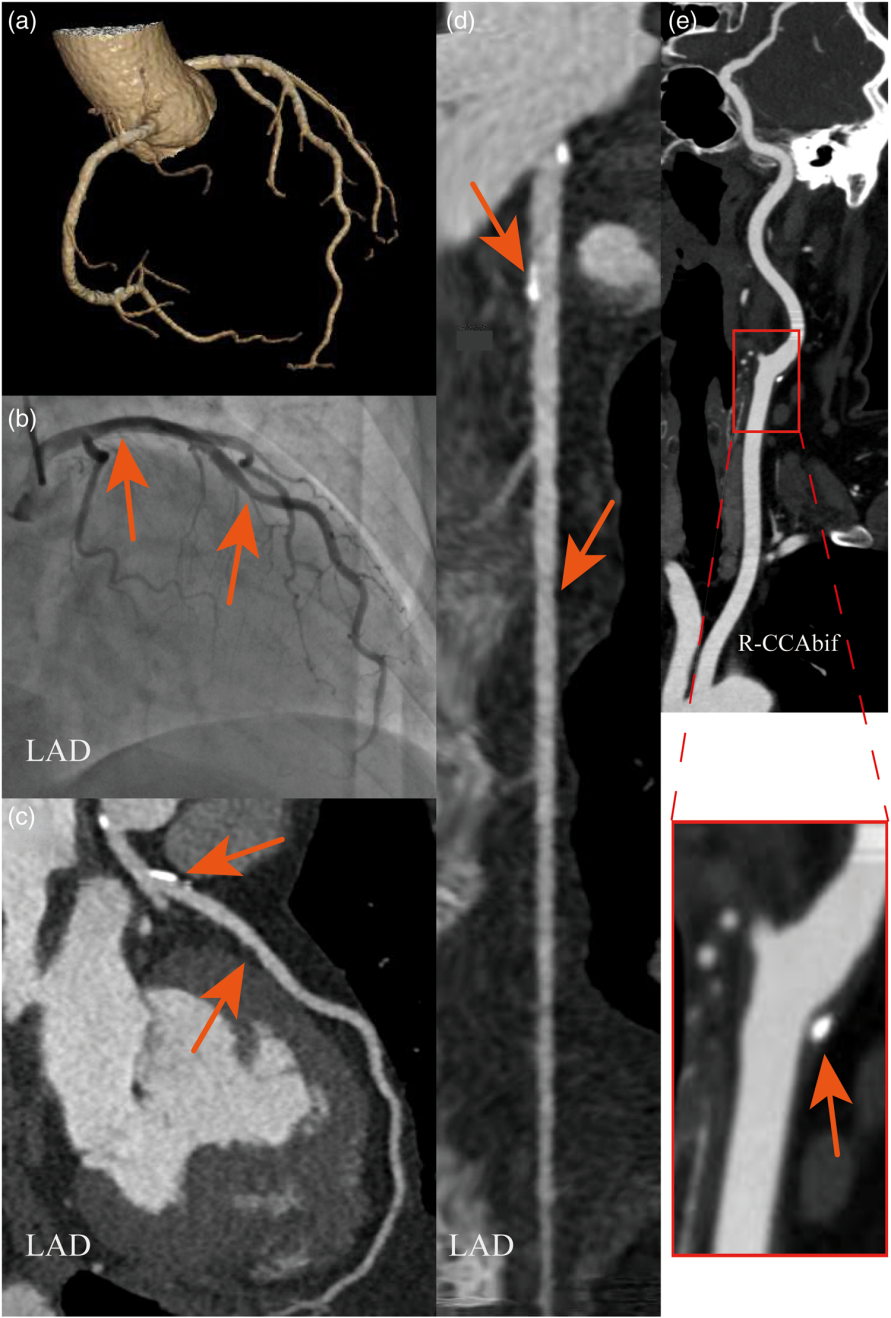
Images of VR (a), DSA (b), coronary CTA (c, d), and craniocervical CTA (e), for a patient performed combined coronary and craniocervical CTA with supported bridge position. A 64‐year‐old woman presented with symptoms of chest tightness and pain. The coronary CTA (c, d) reveals calcified plaques in the LM and LAD, with slight luminal narrowing. The coronary DSA (b) exhibits plaques in the LM and mild narrowing in the LAD, consistent with the findings in coronary CTA. Craniocervical CTA (e) shows calcified plaques at the $R-{\mathrm{CCA}}_{\mathrm{bif}}$. ${\mathrm{CCA}}_{\mathrm{bif}}$, the common carotid artery at the bifurcation; DSA, digital subtraction angiography; LAD, left anterior descending; LM, left main; VR, volume rendering.
